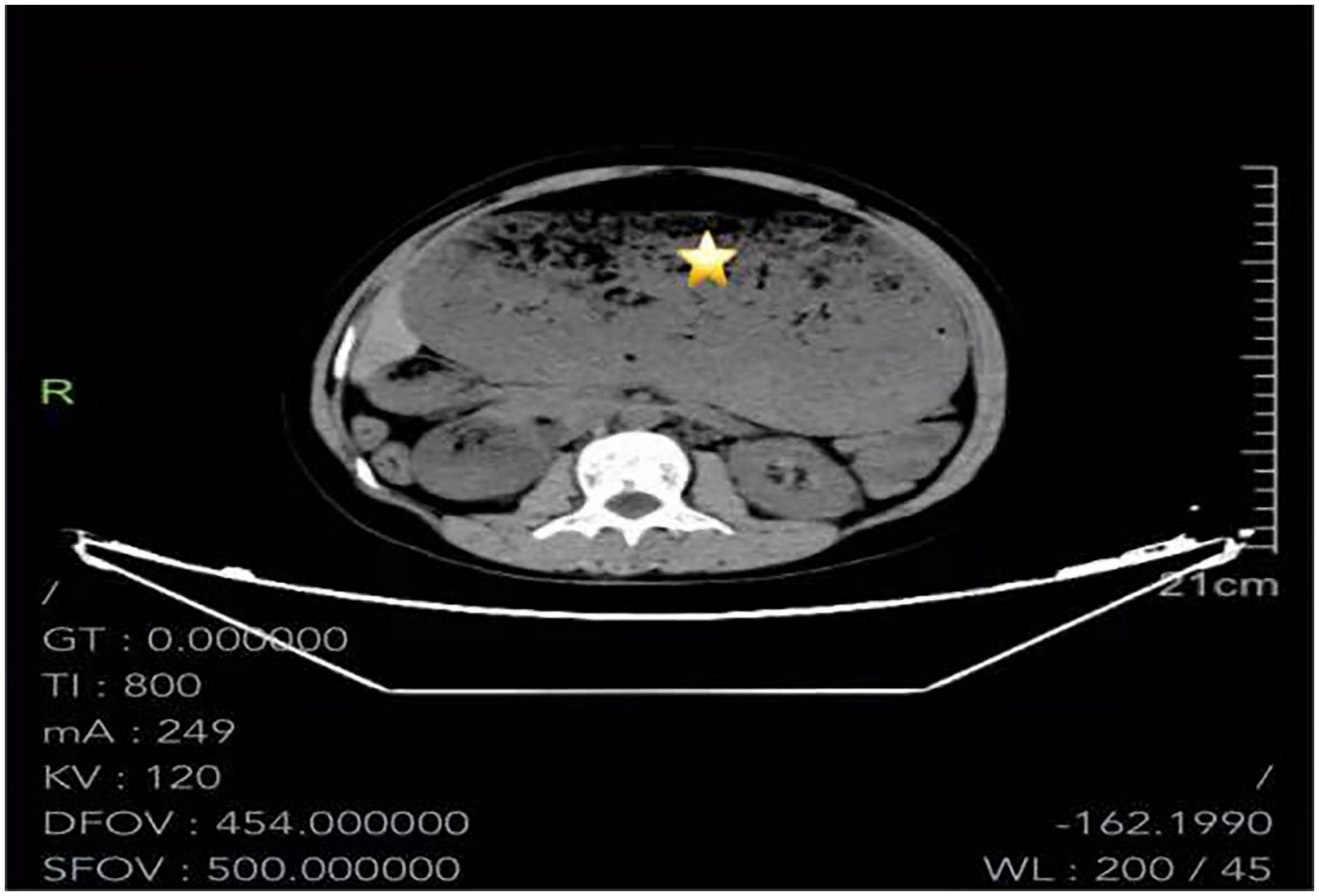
Abdominal CT scan shows that the enlarged stomach is filled with a large amount of gas and food residues.
